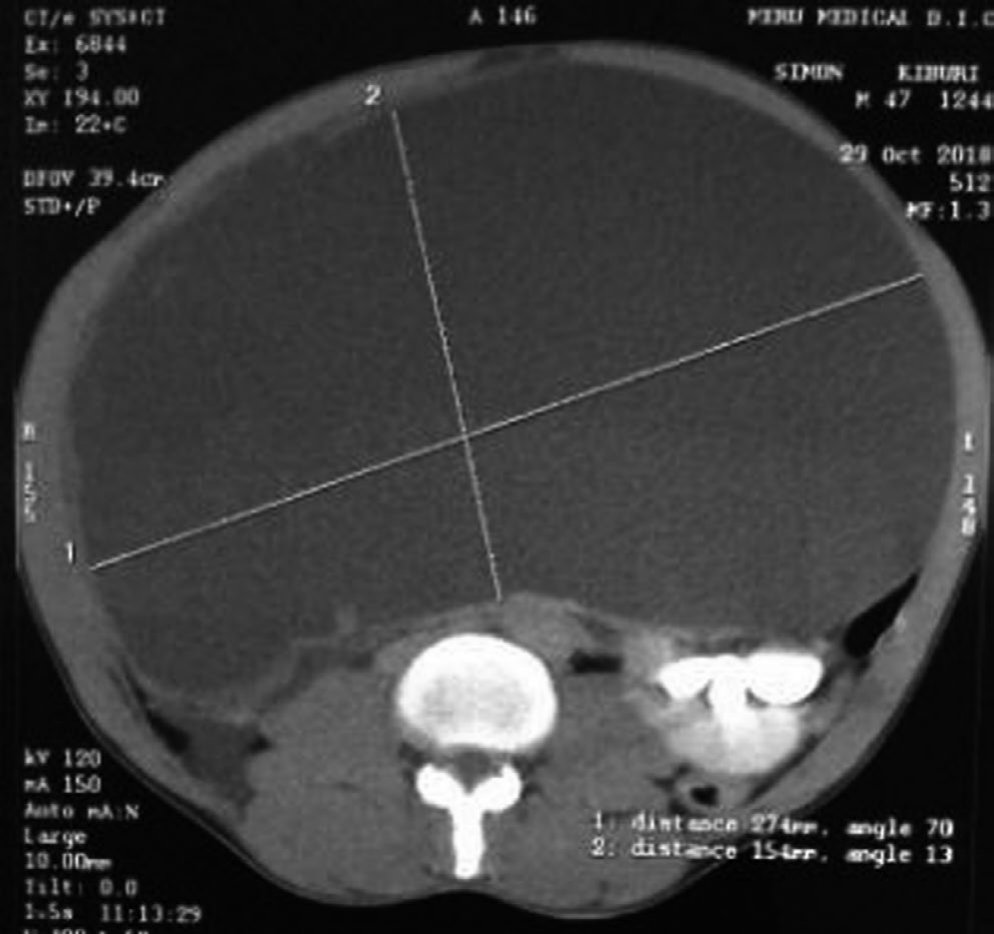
Abdominal computed tomography scan showing the extent of the tumor, coronal view
